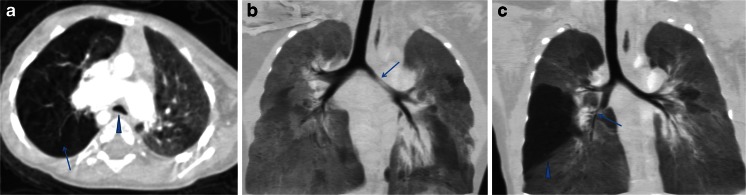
Multi-slice CT findings of location of tracheobronchial compression. a Absent pulmonary valve syndrome with tetralogy of Fallot in a 10-month-old girl. Axial multi-slice CT image demonstrates carina and right main bronchus compression; the carina is shaped like an inverted “V” (*arrowhead*). Right upper lobar emphysema is present (*arrow*). b Absent pulmonary valve syndrome with tetralogy of Fallot in a 13-month-old boy. Multi-slice CT coronal minimum-intensity projection reconstruction demonstrates moderate left bronchial stenosis (*arrow*). c Absent pulmonary valve syndrome with tetralogy of Fallot in a 3-month-old boy. Multi-slice CT coronal minimum-intensity projection reconstruction demonstrates right intermediate bronchial stenosis (*arrow*) and right middle lobe obstructive emphysema (*arrowhead*)
